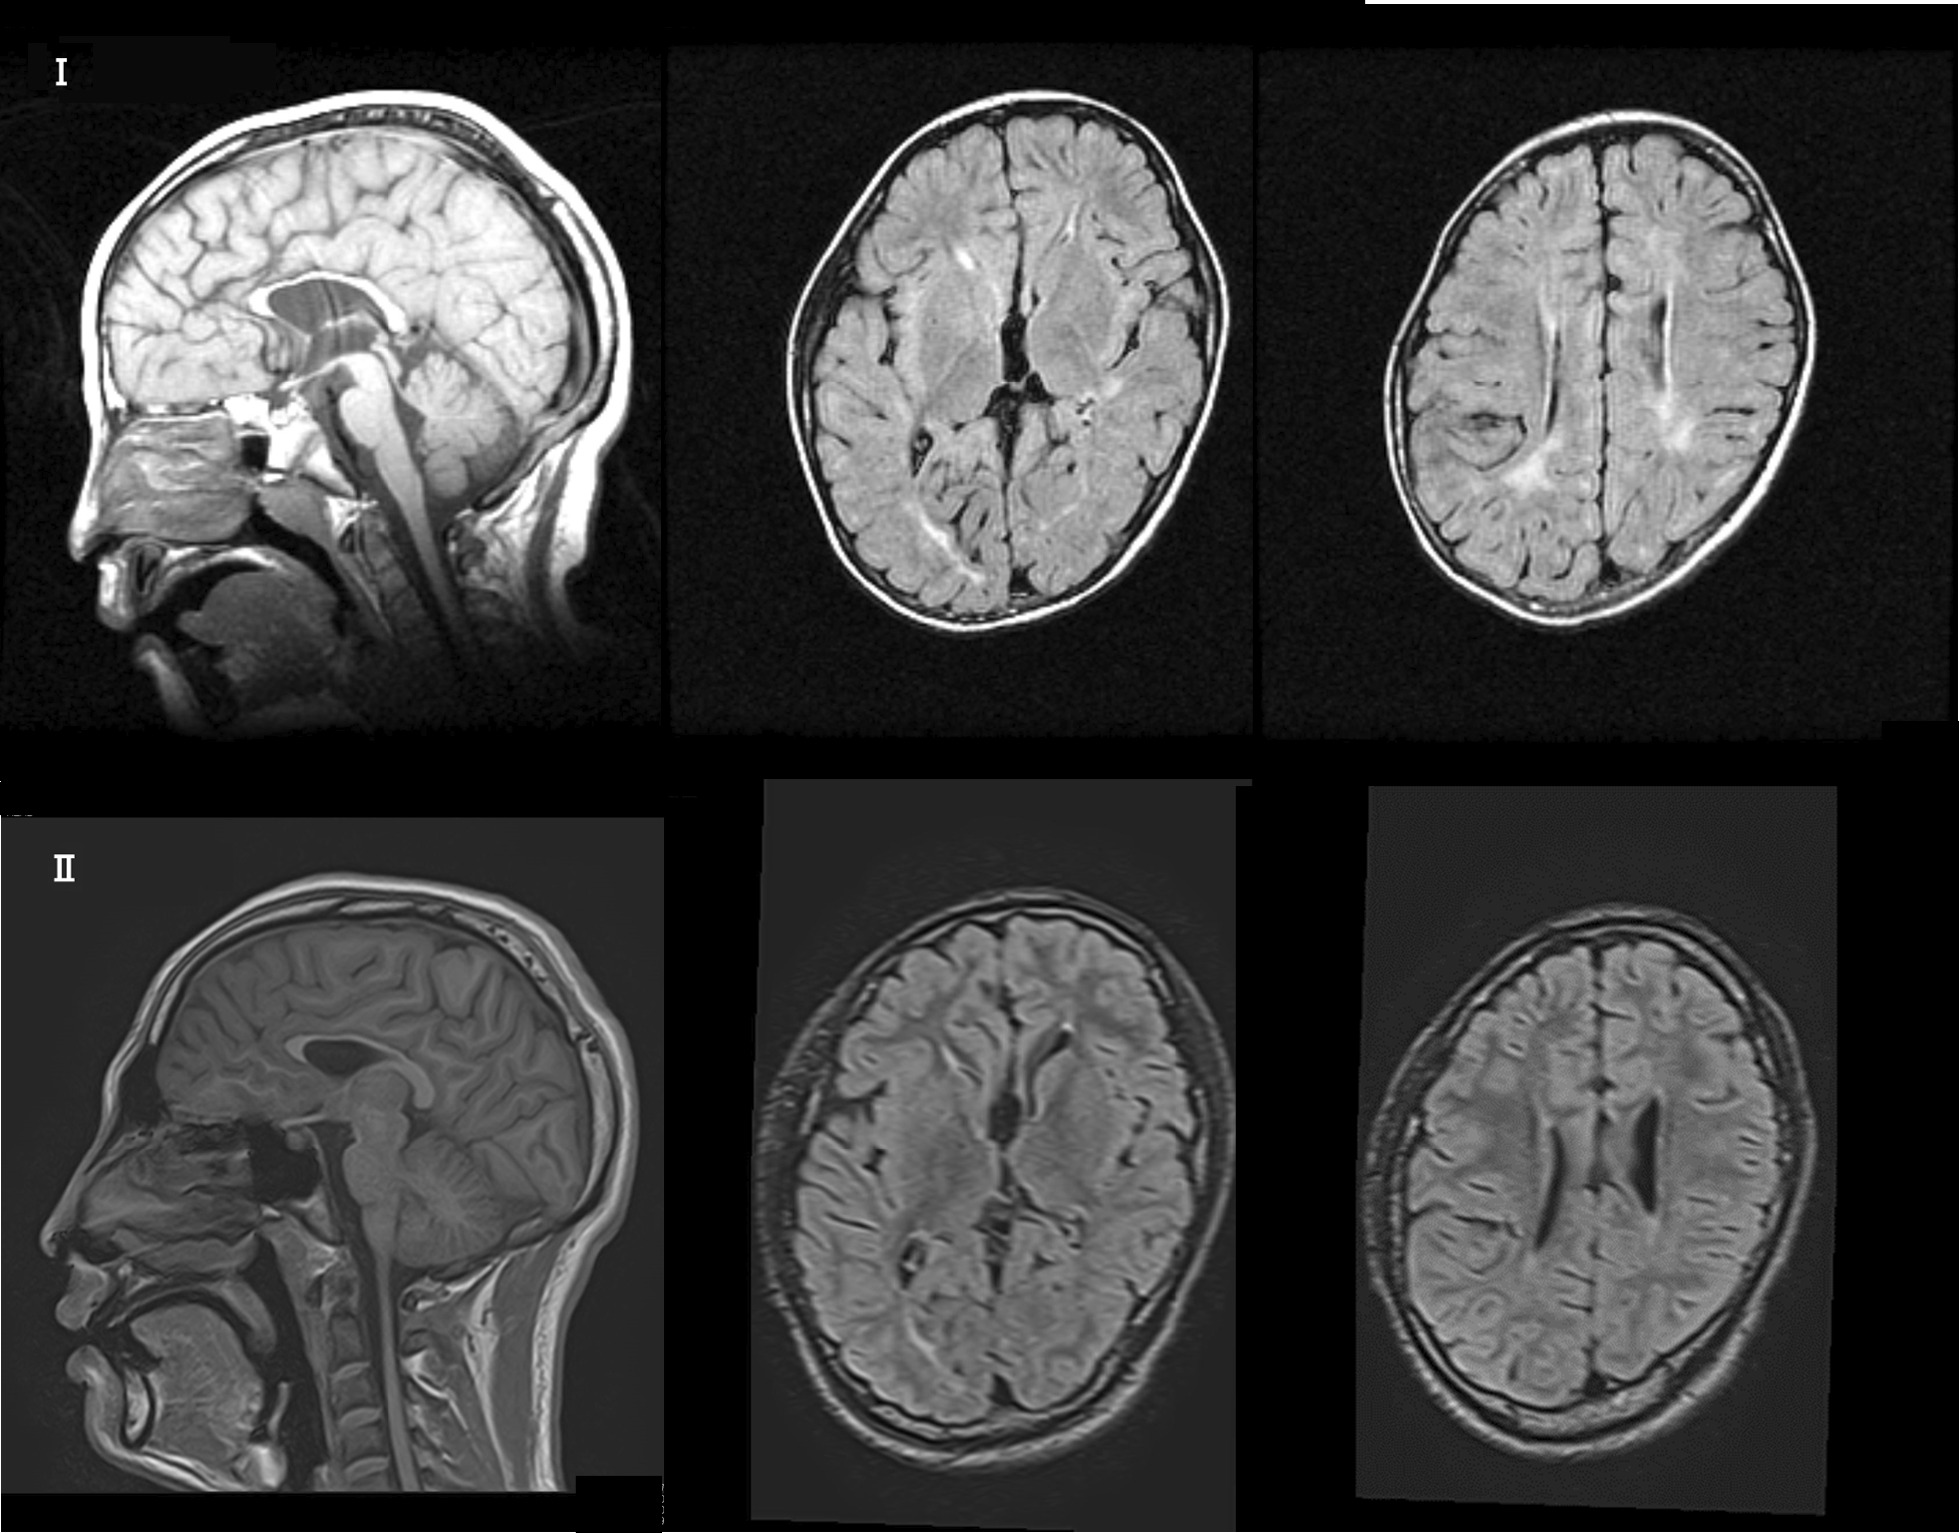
I Magnetic resonance imaging (MRI) image of patient at 3 years of age: T1-weighted and FLAIR images exhibit microcephaly; Verga’s cavity; hypoplasia of the corpus callosum, cerebellum, and brain stem; linear hyperintensity in the deep white matter; and unclear hypersensitivity in the cortex and white matter. II MRI image at 17 years of age: T1-weighted and FLAIR images show microcephaly; Verga’s cavity; hypoplasia of the corpus callosum, cerebellum, and brain stem; and decreased white matter capacity. The high intensity in the deep white matter observed during infancy was lowered
